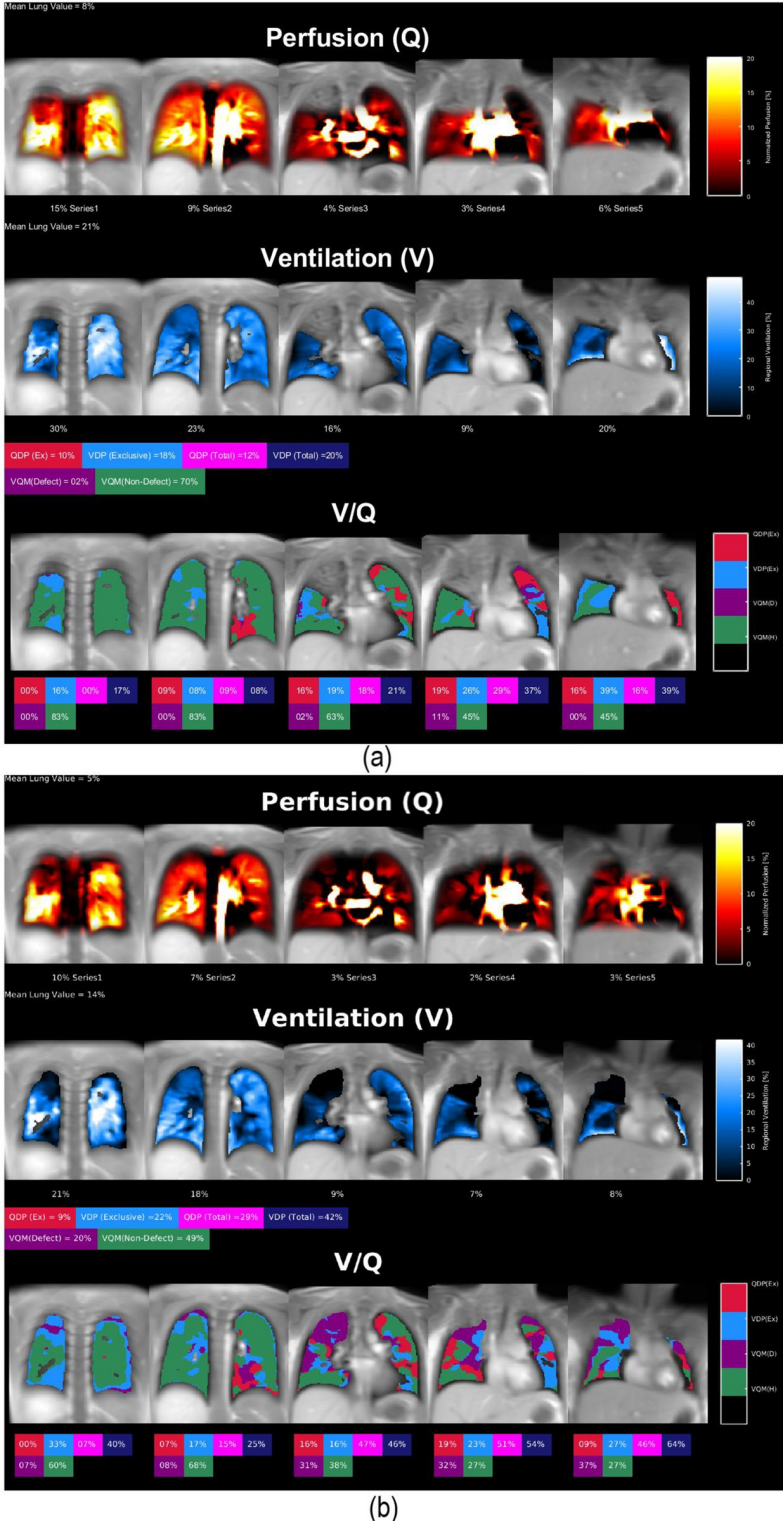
Impact on functional lung values caused by the improvements in segmentation performance: (a) shows the Perfusion (Q) and Ventilation (V) maps based on the segmentation from CNN_Bal/NoCons_: trained with balanced augmentation and without artificially-generated consolidations. (b) shows Q and V maps based on the segmentation from CNN_Bal/Cons_ (proposed method): trained with balanced augmentation with artificially-generated consolidations. Notice, that ventilation and perfusion parameters change essentially when whole lung parenchyma including the real consolidation is correctly segmented using CNN_Bal/Cons_. Ventilation and perfusion defect percentage maps increased substantially (from 20% up to 42% for ventilation and from 12% to 29% for perfusion) with the proposed CNN network. The images were created with Phase Resolved Functional Lung MRI^3^ (PREFUL) method.
